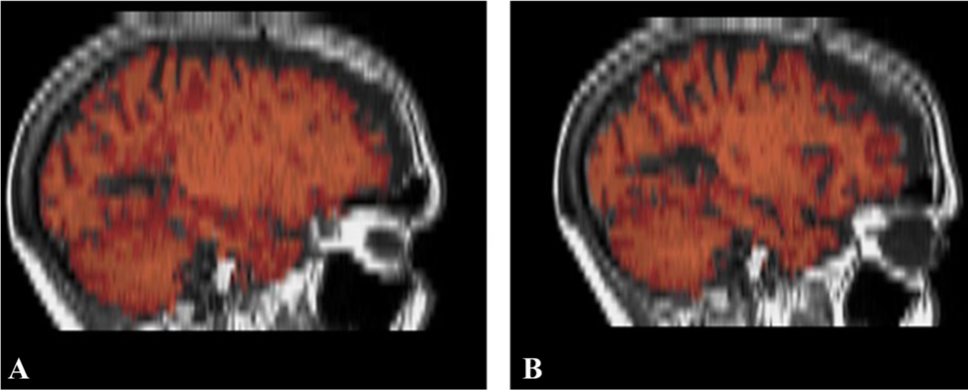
The MRI post-analyisis detected a reduction of 2 % in total brain volume (TBV) between before (a: TBV = 1246 ml) and after treatment (b: TBV = 1228 ml), as assessed by the Siena tool of FSL software
